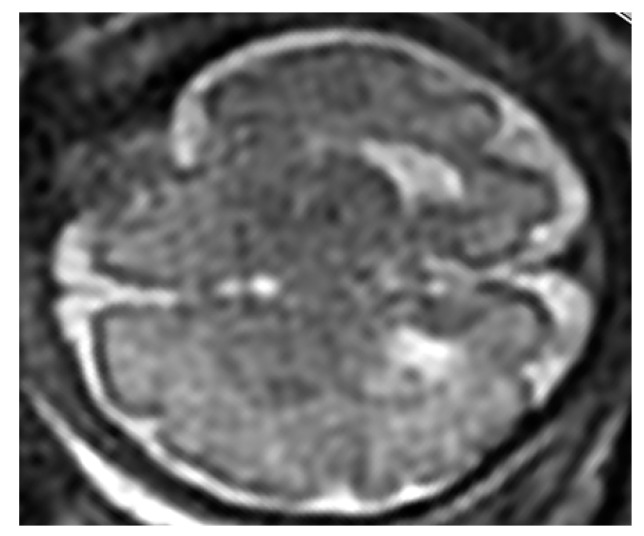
MRI picture of lissencephaly.
